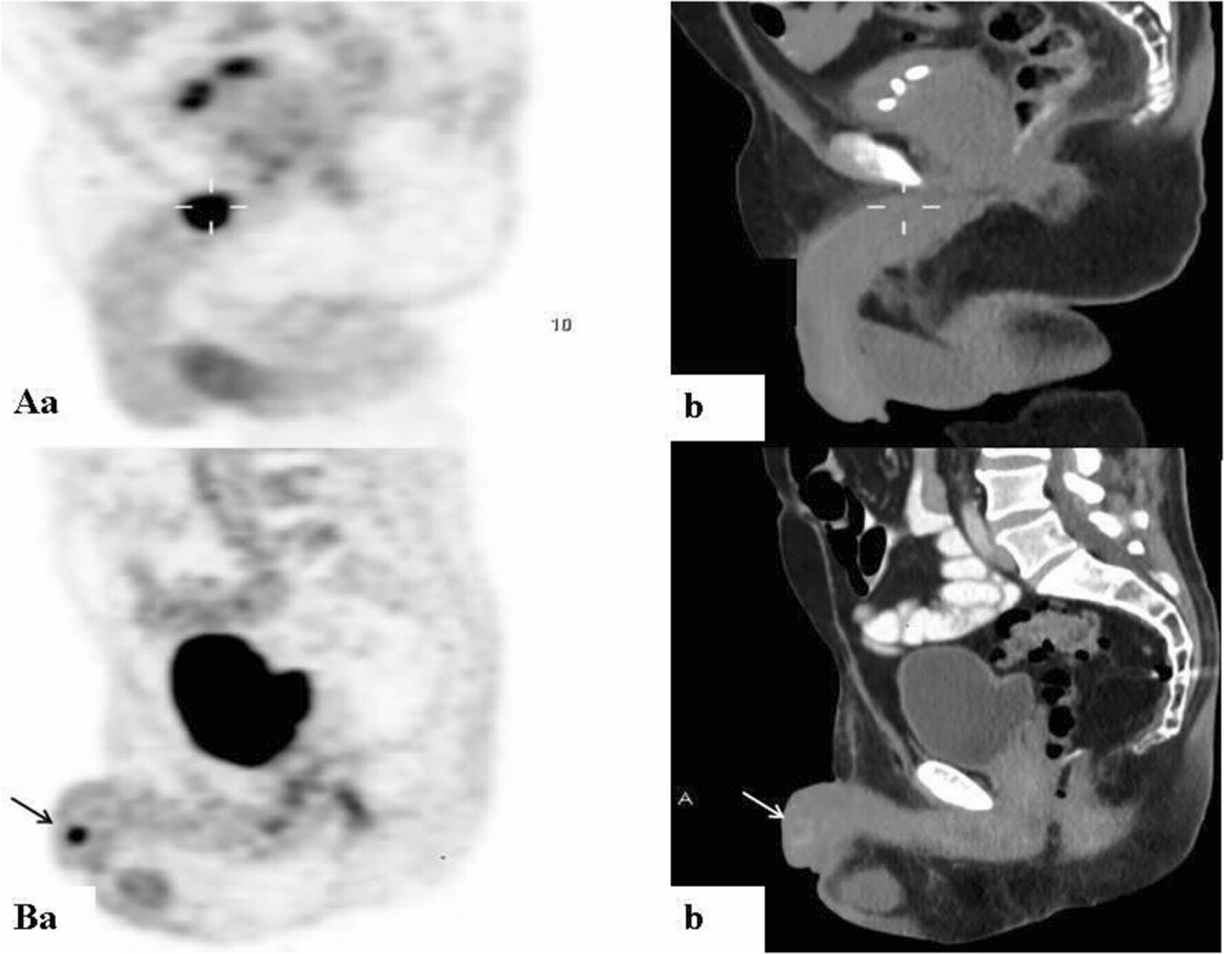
18F-FDG scans demonstrating focally increased uptake at different places along the penis on PET (a) and CT (b) sagittal slices. (A) An 84-year-old man with metastatic high-grade urothelial carcinoma (patient #22, Table 1). (B) A 49-year-old man with metastatic adenocarcinoma of the lung (patient #20, Table 1).
